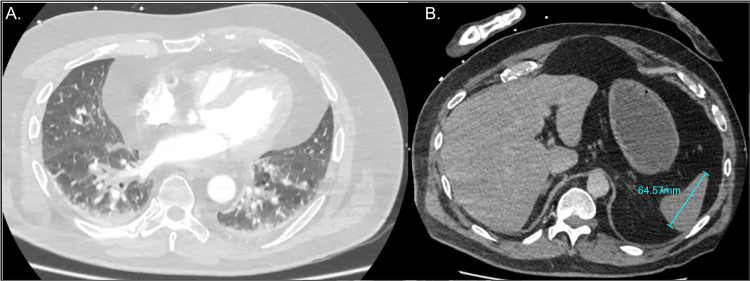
Total body scan at admission A. Axial chest CT scan showing non-specific ground-glass opacities associated with bibasal atelectasis. B. Contrast-enhanced abdominal CT demonstrating a small spleen measuring 64.57 mm in length.
